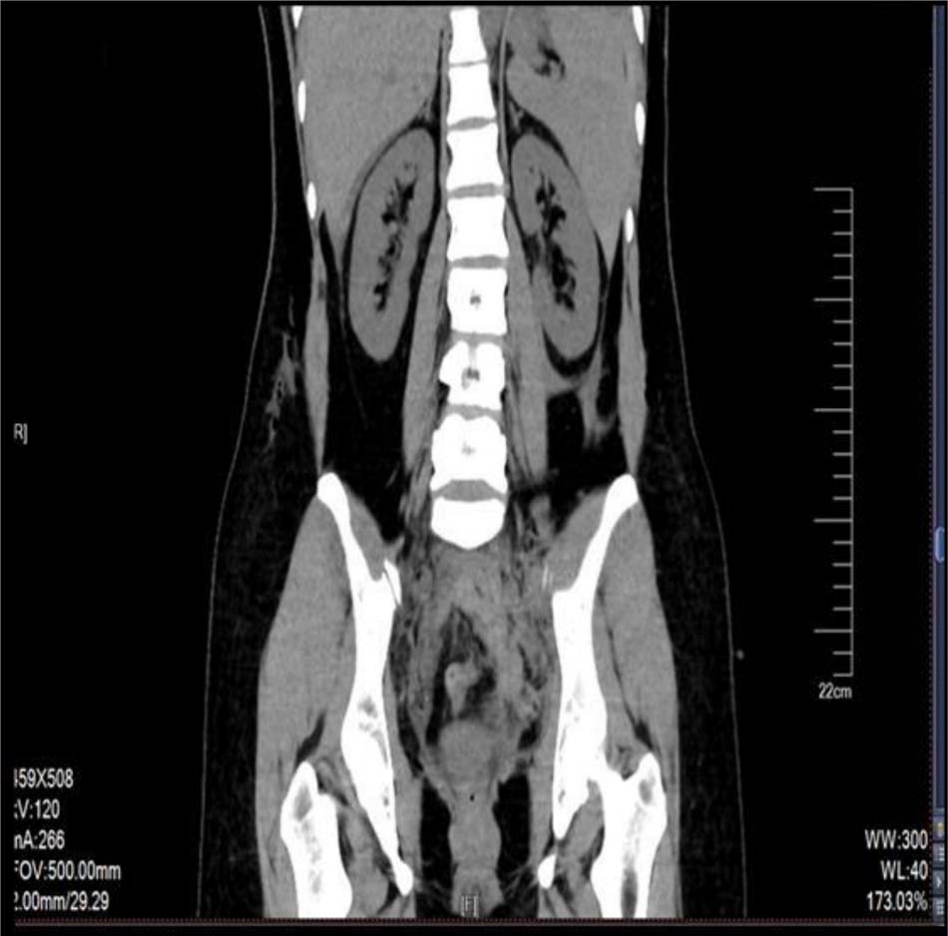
CT scan of the retroperitoneum hematoma.
